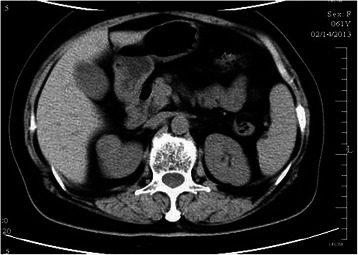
CT showing the presence of homogeneity and well-defined solid renal masses.
